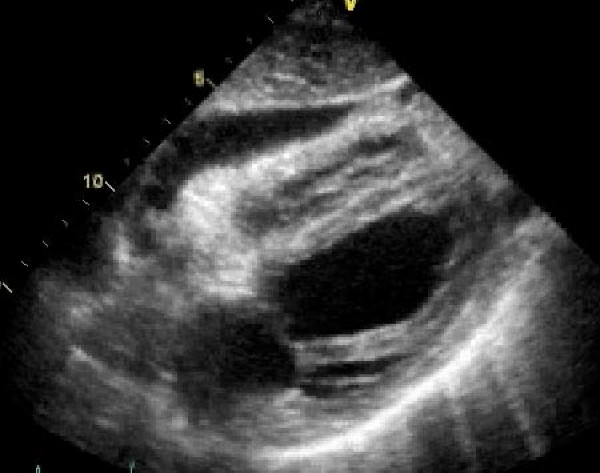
A transthoracic echocardiogram 4 chamber view from subxiphoid approach representing a 25 mm echolucent region adjacent to the right ventricular free wall and right atrium, presumed to be due to a pericardial effusion.
